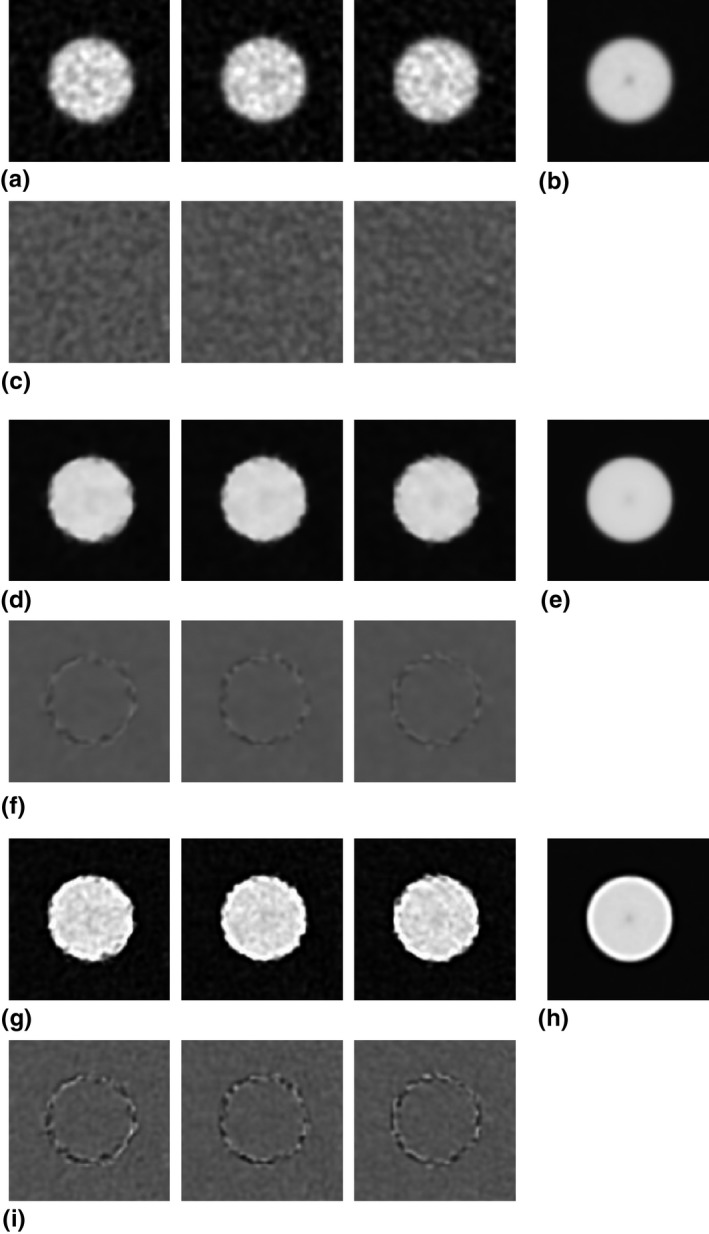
(a) Three example computed tomography (CT) cross‐sectional images of a cylindrical object reconstructed using the filtered back projection (FBP) algorithm. (b) The mean image obtained by averaging all FBP images. (c) The images obtained by subtracting the mean image (b) from each slice image (a). (d–f) Results obtained using IMR *Body Routine* level 3. Images are analogous to those in (a–c). (g–i) Results obtained using iterative model reconstruction *SharpPlus* level 3. The images are analogous to those in (a–c). The window settings are WL/WW = −225/310 HU for images in (a, b, d, e, g, h), and 0/200 HU for images in (c, f, i).
